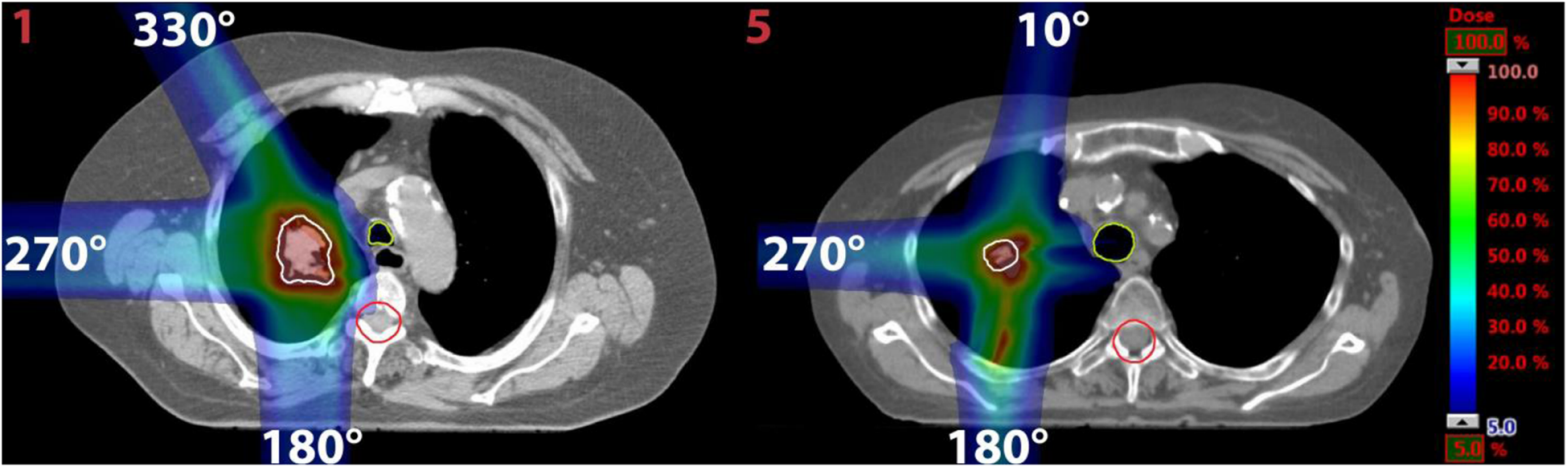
CT slices of the patients 1 and 5 (marked in red numbers) with the dose distributions for the optimized IMPT plans. The CTV is marked in white, trachea in light green and spinal cord in red. On the right a color bar is given indicating the relative dose
